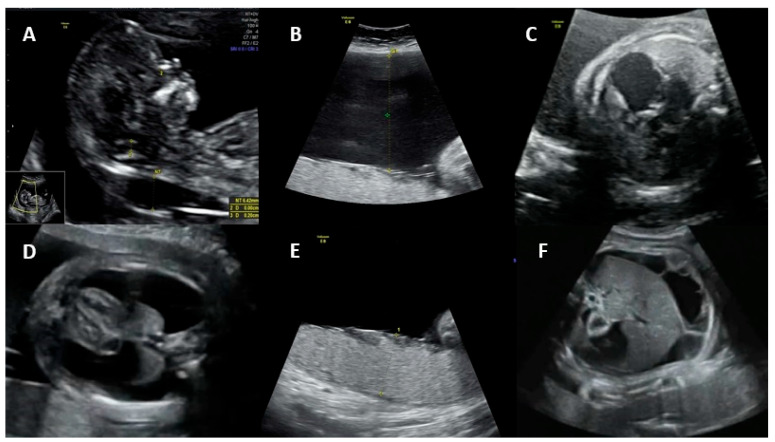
Main ultrasonographic findings of intrauterine parvovirus B19 infection: (A) increased nuchal translucency, (B) polyhydramnios, (C) cardiomegaly, (D) pleural effusion, (E) placentomegaly, and (F) ascites.
